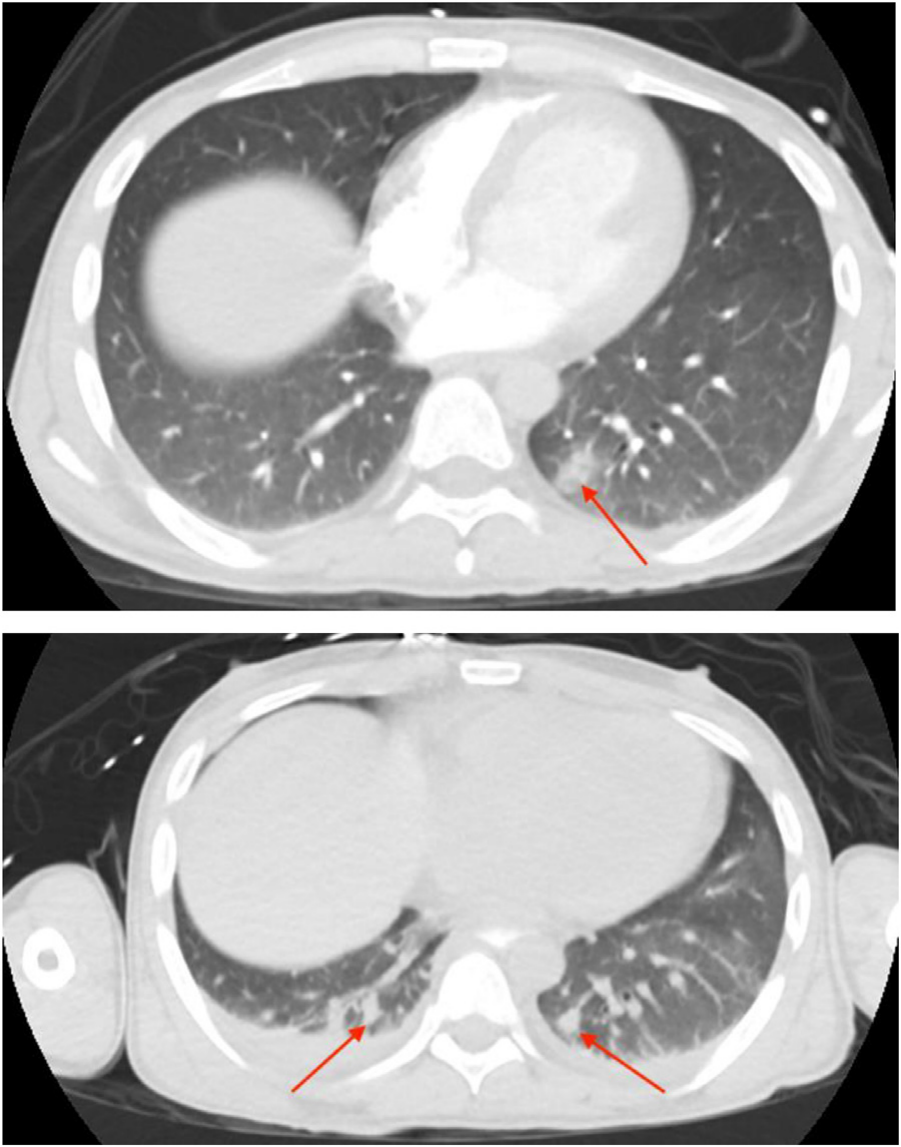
CT of the chest with contrast near the time of admission (A) and a month later (B) demonstrates patchy opacities within the left lower lobe which become more nodular and bilateral distribution (red arrows) on the subsequent examination consistent with the patient's multifocal pneumonia.
